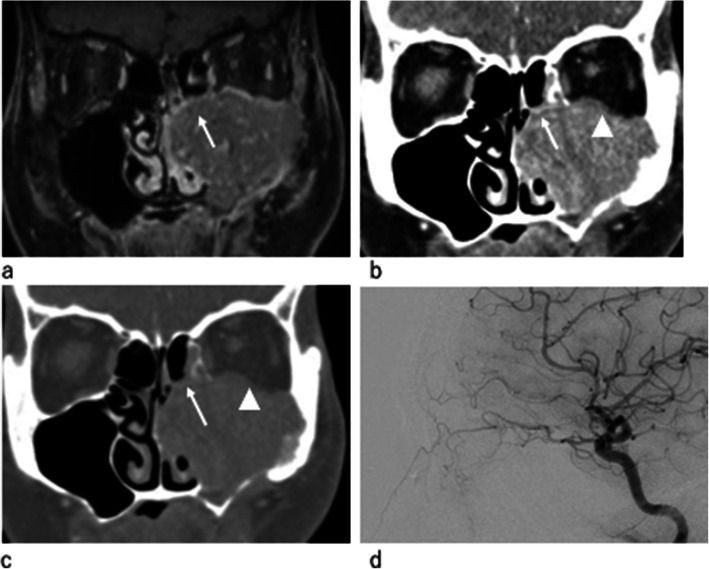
Patient showed swollen extension of MSC in the ethmoid sinus on the coronal section of fat-suppressed T1 weighted image (a; white arrow); however, there was no borderline bone destruction on the coronal section of contrast-enhanced CT (b, c; white arrow), and it was determined that there was no invasion. In this case, it was difficult to determine the presence or absence of invasion into the ethmoid sinus on MRI, and CT was referred to determine the absence of bony destruction. (b, c; arrow) Infiltration into the orbital floor with bony destruction was noted. (d) A lateral view of the left internal carotid angiogram showed no enhancement of MSC from the ophthalmic artery
